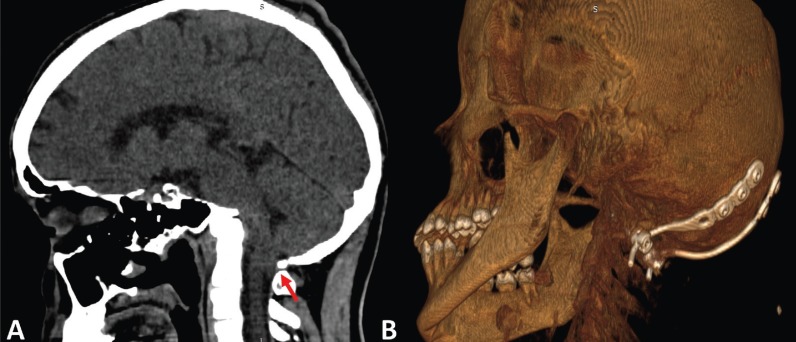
ACM-I male patient. A. The computed tomography (CT) scan demonstrates the cerebellum herniation (red arrow). B. Post-operative CT scan of the same patient. Stabilization of the cervical vertebrae was also required (arthrodesis).
